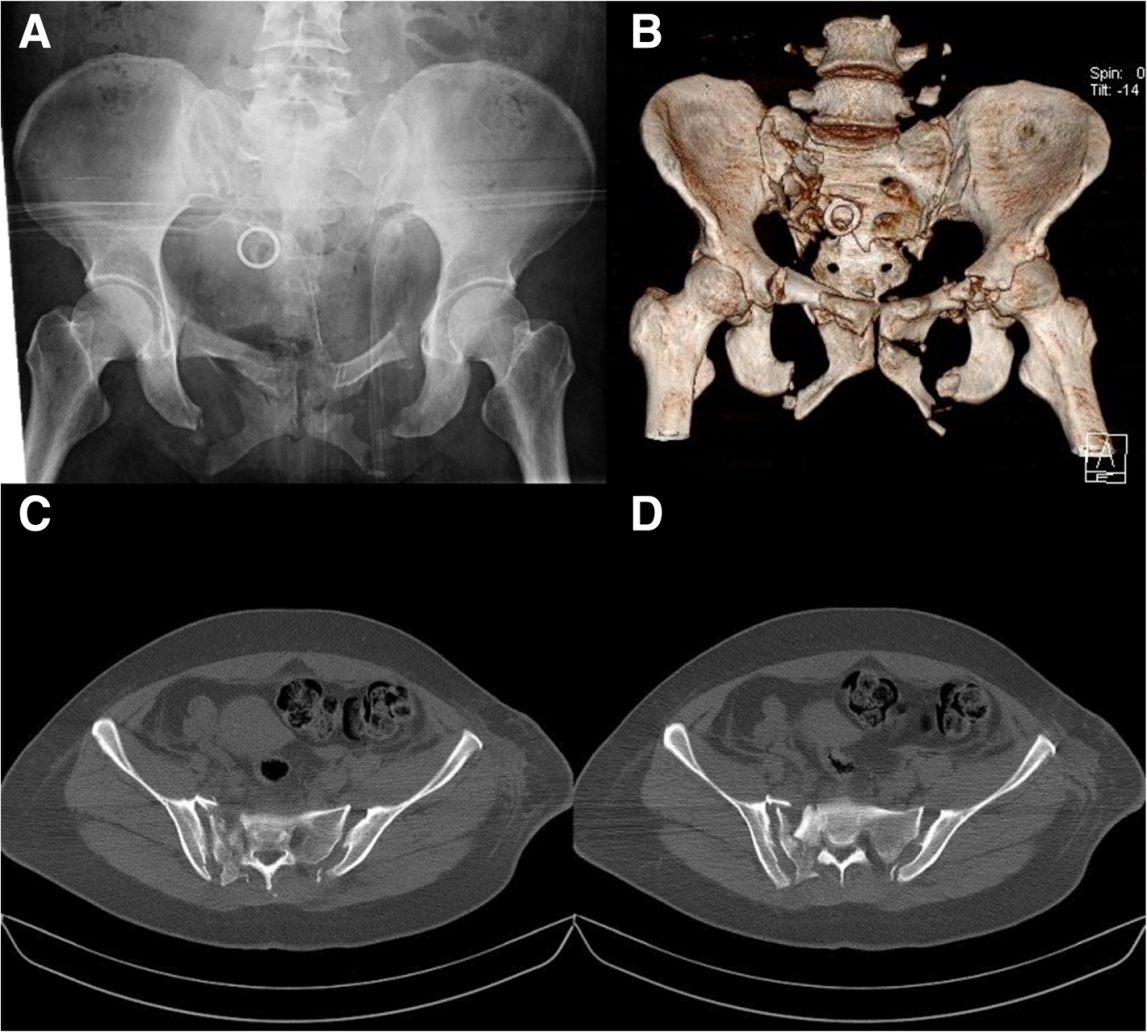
a–d AP view and CT 3D reconstruction of pelvic fracture showed type C3 (Tile classification) fracture with Denis II fracture and compressed fragment in S1 foramina
